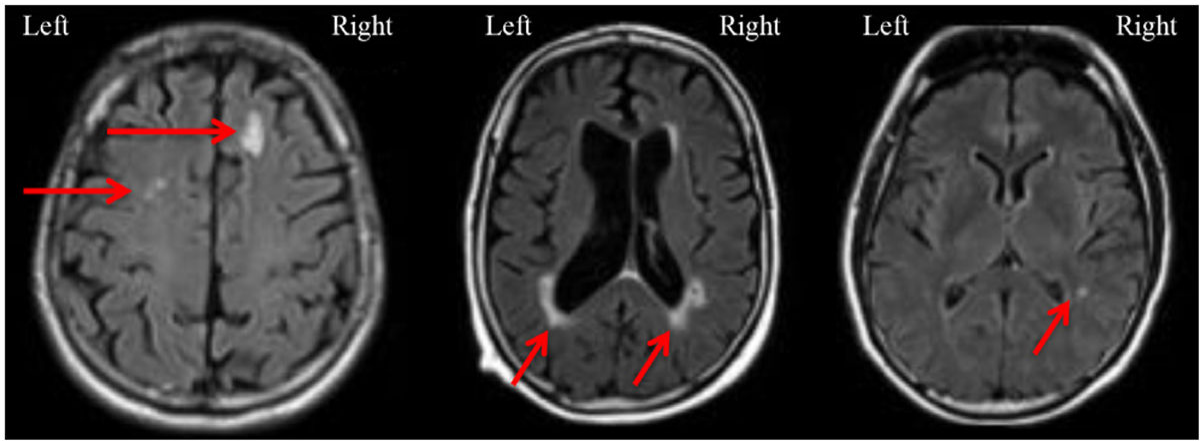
WMH on FLAIR. This figure presents FLAIR image examples of WMH in the frontal region (top), the parieto-occipital region (middle), and temporal region (bottom). Right and left are indicated for orientation. Red arrows indicating WMH on the images.
